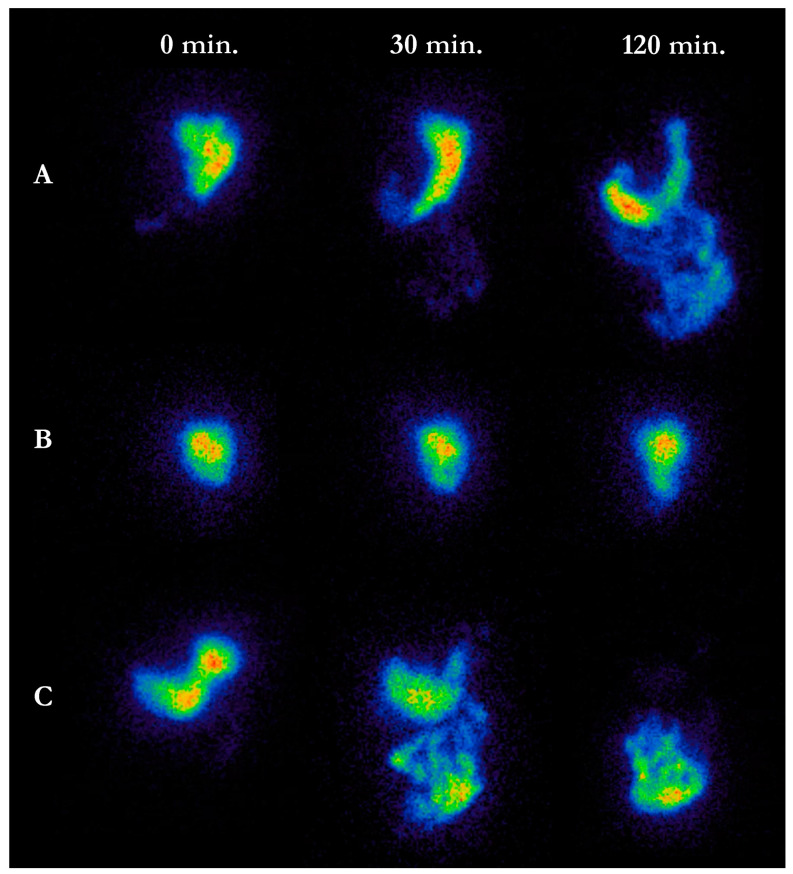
Gastric scintigraphy images at 0, 30 and 120 min after ingestion of a radioactive solid meal. (A). Healthy control with normal gastric emptying time. (B). Parkinson’s disease (PD) patient with severely delayed gastric emptying time compatible with gastroparesis. (C). PD patient with rapid gastric emptying suggestive of ”gastric dumping” (compare the image taken at 30 min. to the image from A taken at 120 min.).
